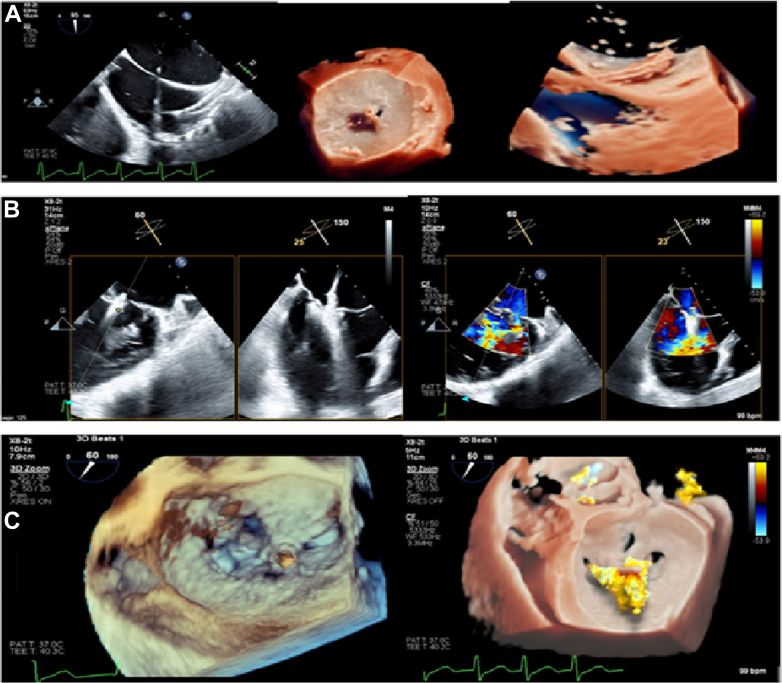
Echocardiographic Images Showing Different Stages of the Initial XTW MitraClip Placement (A) Two- and three-dimensional images showing transseptal puncture during TEER procedure: (B) Two-dimensional and color Doppler images showing successful deployment of the first XTW MitraClip with moderate residual mitral regurgitation. (C) Three-dimensional images showing successful deployment of the first XTW MitraClip with moderate residual mitral regurgitation. TEER = transcatheter edge-to-edge repair.
